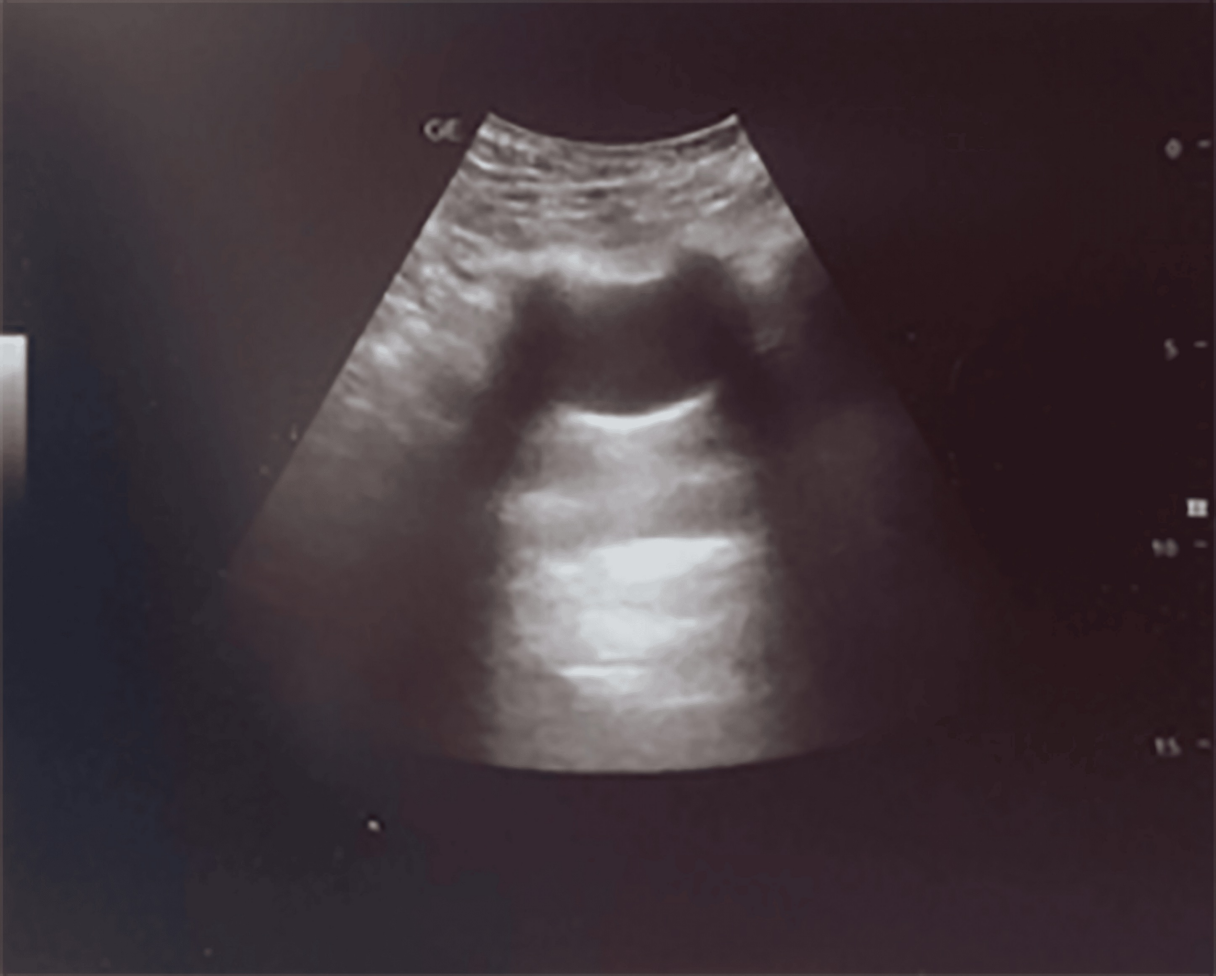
Chest ultrasound of pulmonary cyst.
